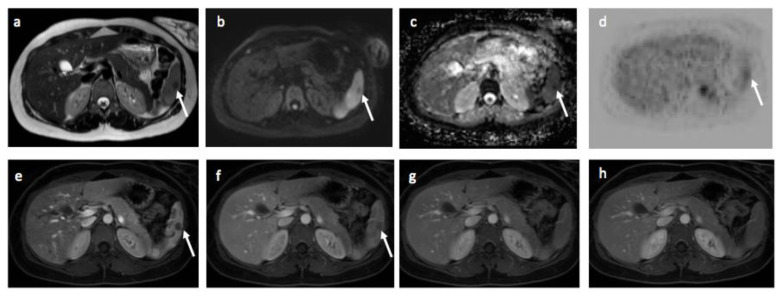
Images of a 24-year-old female patient with Hodgkin’s lymphoma highlighting extra-nodal perceptual error on ^18^F-FDG PET-CT. Splenic involvement was considered positive on WB-MRI and negative on ^18^F-FDG PET-CT. Following the consensus review, the panel corrected the disease site to be positive on ^18^F-FDG PET-CT and subsequently, the enhanced reference standard was positive for splenic involvement. (a) T2-TSE, (b) DWI b_1000_, (c) ADC map, (d) ^18^F-FDG PET, (e) DCE spleen: early-arterial phase, (f) DCE spleen: mid- arterial phase, (g,h) DCE spleen: late-arterial phase. Arrows showing a focal lesion in spleen.
